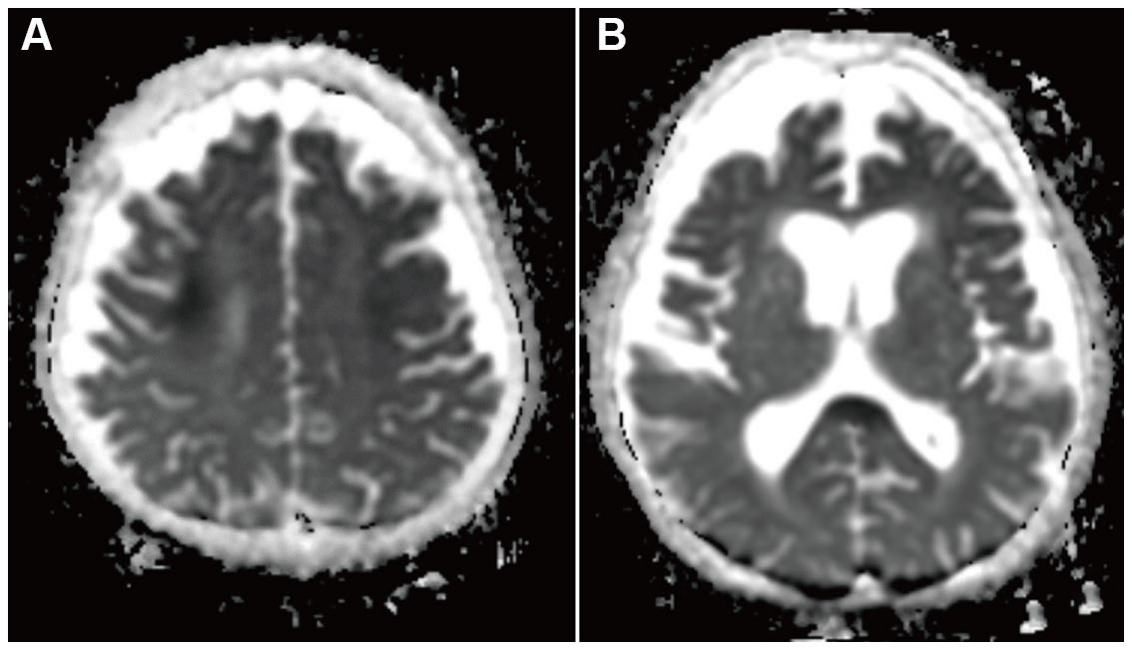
Apparent diffusion coefficient maps brain MRI on day 2 of admission. Hypointensity was in the bilateral deep white matter (A) and the corpus callosum (B). This hypointensity was consistent with DWI hypointensity areas.
